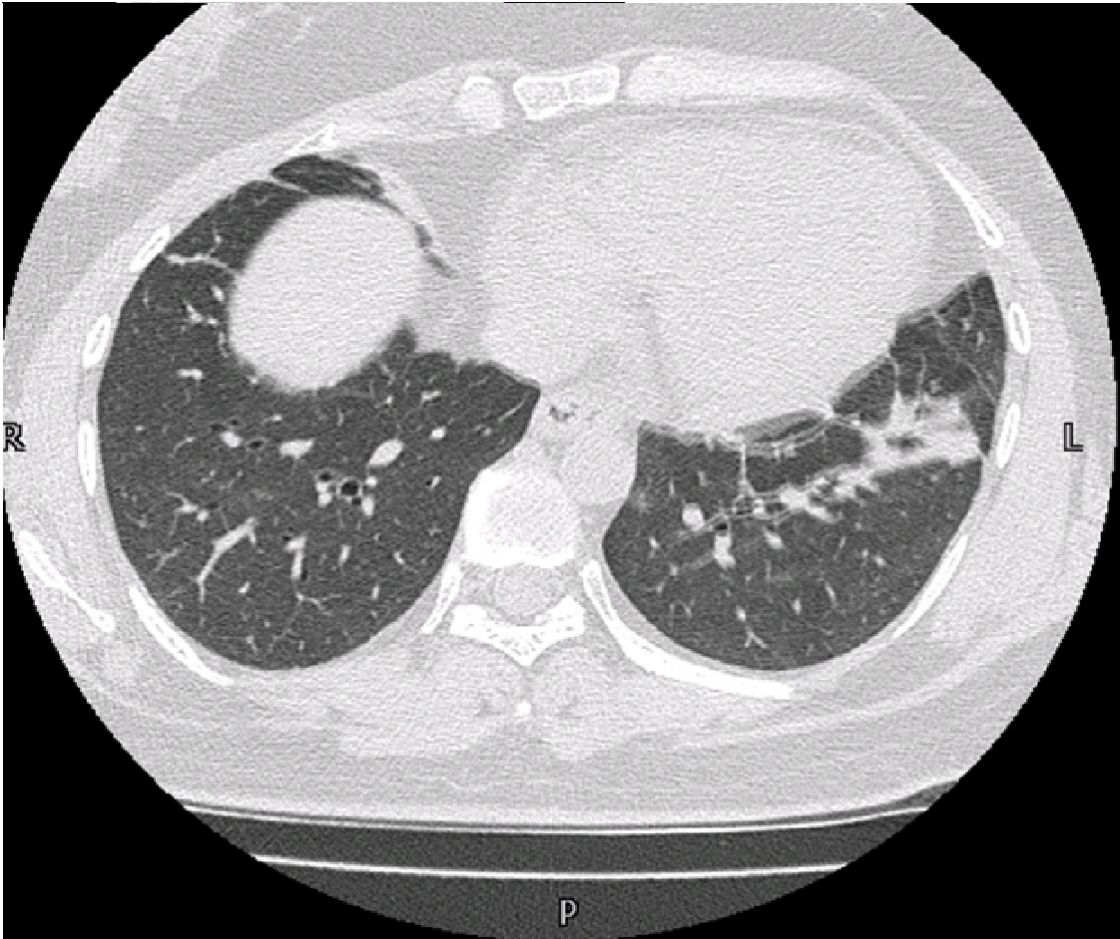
High-resolution computed tomography (HRCT) showing fibrosis and scarring more marked on the left than on right
